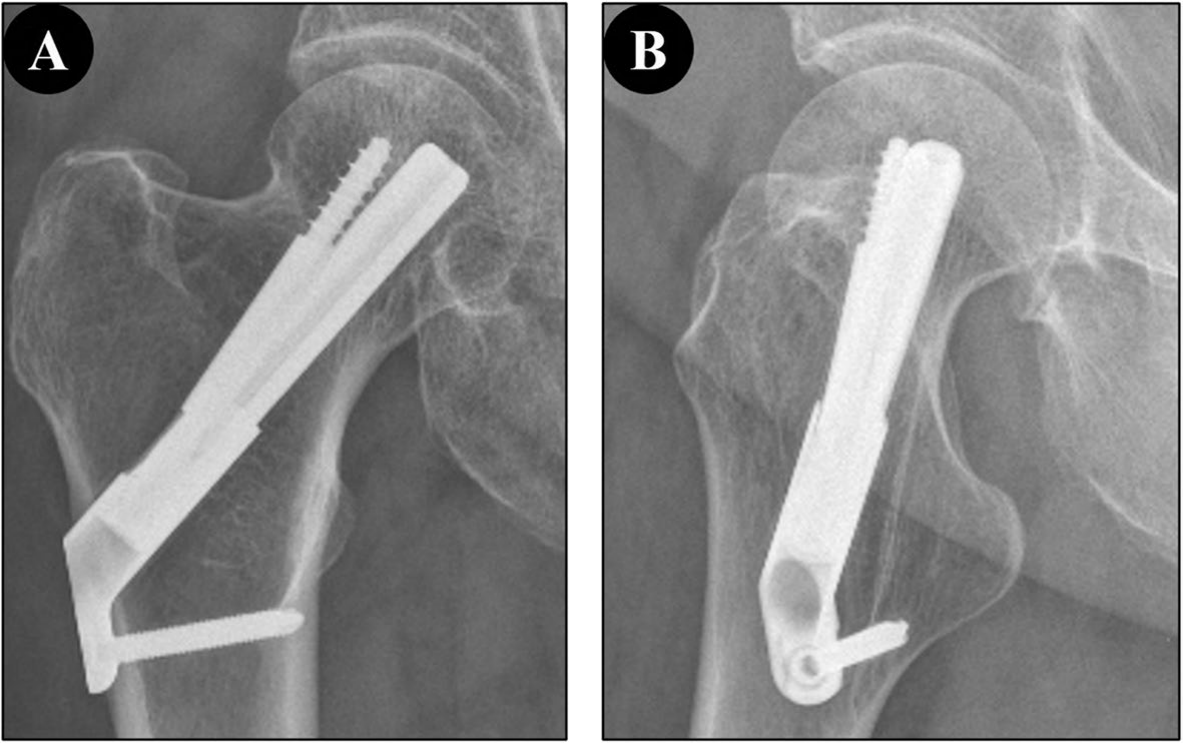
A and B Hip joint frontal and axial X-rays treated with FNS
